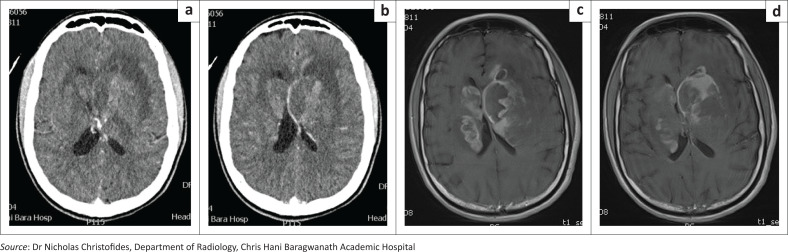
Typical CT scan findings from a patient in this case series. (a) Selected axial slices of contrast-enhanced CT brain displaying a heterogeneous midline lesion involving bilateral cssaudate nuclei and corpus callosum with associated adjacent vasogenic oedema; (b) Selected axial slices of T1-weighted contrast-enhanced magnetic resonance imaging (MRI) brain confirming the midline lesion with invasion of bilateral basal ganglia, caudate nuclei and corpus callosum. The lesion displays heterogeneous peripheral enhancement and perilesional oedema. There is no associated dural enhancement to suggest dural spread.
